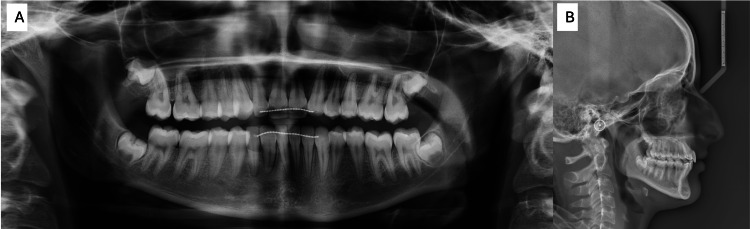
Post-treatment radiographs. The images show (A) post-treatment panoramic and (B) post-treatment lateral cephalogram.
